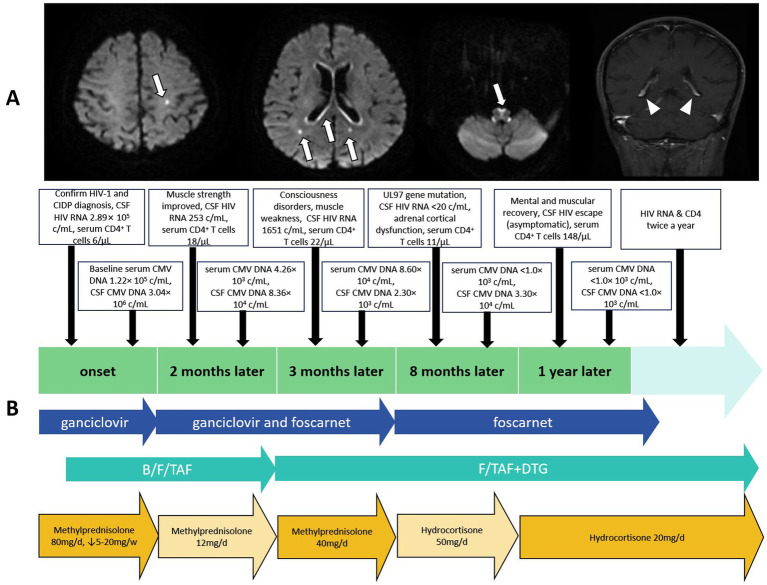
(A) Brain magnetic resonance imaging (MRI) of the patient. Diffusion-weighted imaging sequence revealed high signals in the left half of the oval center, near the posterior horn of the bilateral ventricles, bilateral ventricular walls, and anterior edge of the medulla oblongata (white arrows). The coronal image showed abnormal enhancement of the bilateral ventricles (Triangles). (B) The timeline for treatment adjustment and follow-up of this patient.
